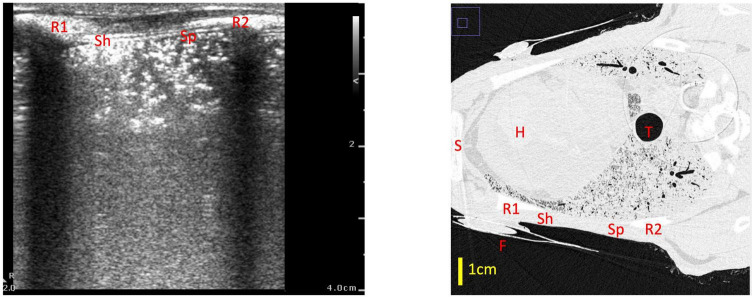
Example of a type 0.5 lung ultrasound image and a slice from the corresponding CT scan, with the medial rib (R1), lateral rib (R2) and fiducial (F) highlighted in both images. For reference, the heart (H), trachea (T) and sternum (S) are also labeled in the CT. The part of the ultrasound image closest to the medial rib (Sh) has a sharper pleural line which appears more superficially on the ultrasound image (at a depth of ∼0.5 cm), which corresponds with a better aerated portion of lung on CT—in this section, the ultrasound beam did not travel far before encountering a large, reflective block of air. The part of the ultrasound image which is closer to the lateral rib (Sp) has a more speckled pleural line, with small sections of the pleural line appearing at various depths from 0.5 to 2 cm. This corresponds with a more poorly aerated segment of lung on CT. In this section, the unaerated portions of lung form liquid/tissue channels which the ultrasound beam can travel through, until it gets reflected by small pockets of air which are present at various depths below the pleural line, creating the speckled appearance in the ultrasound image.
